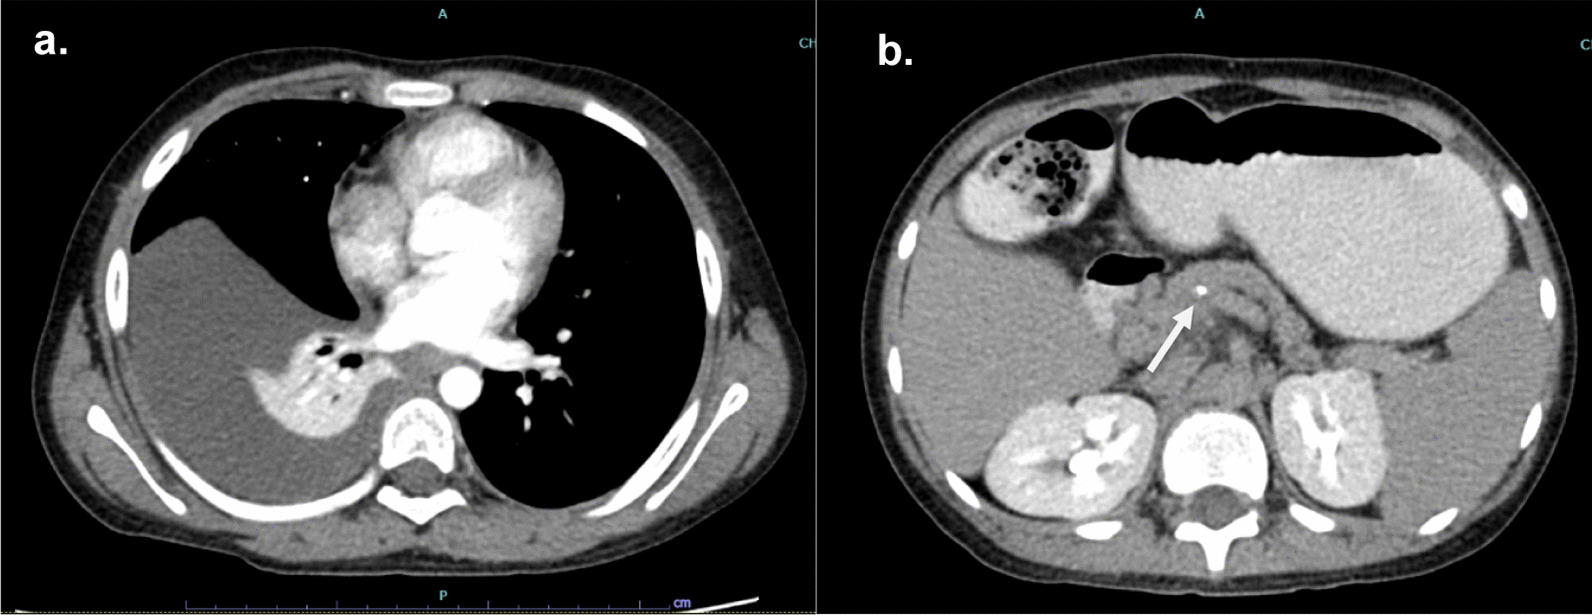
Initial CT scan reveals a pleural effusion and b calcification in the head of pancreas with dilatation of pancreatic duct (arrow)
